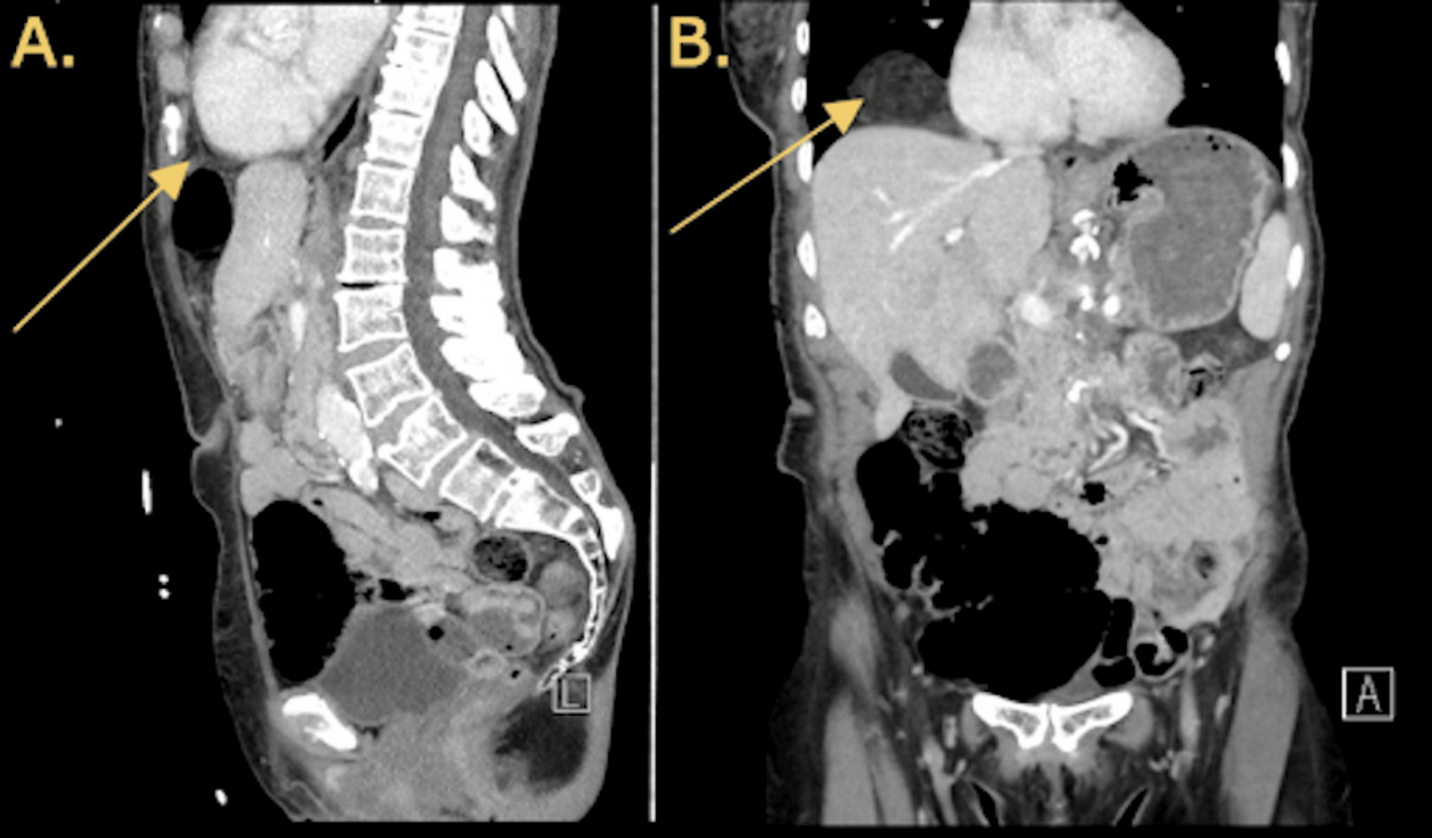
CT imaging of the abdomen and pelvis demonstrating Morgagni hernia findings A) Sagittal CT findings with the arrow indicating a right anterior diaphragmatic defect with a nondilated loop of transverse colon herniating into the thoracic cavity. B) Coronal CT findings with the arrow indicating a right anterior diaphragmatic defect with a nondilated loop of transverse colon herniating into the thoracic cavity CT: computed tomography
